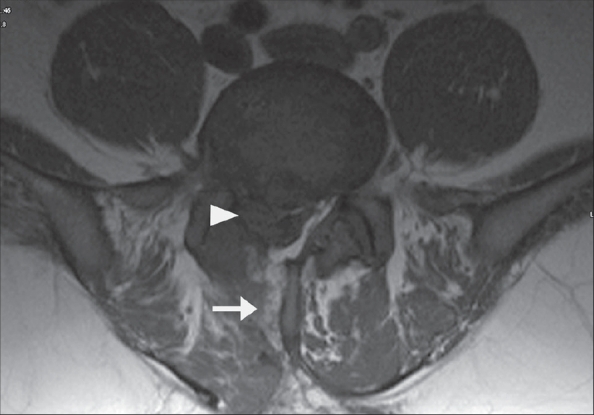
Axial MR image of a patient in the revision category - MRI showing track of previous procedure (arrow) and sequestered fragment in canal (arrow head)
